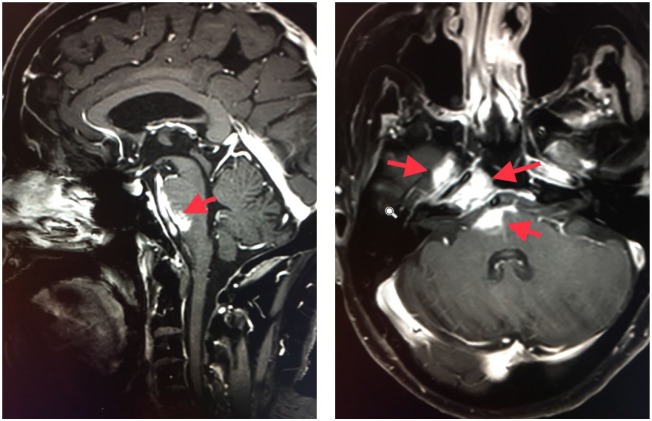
Magnetic resonance imaging (MRI) of metastatic spindle cell squamous cell
